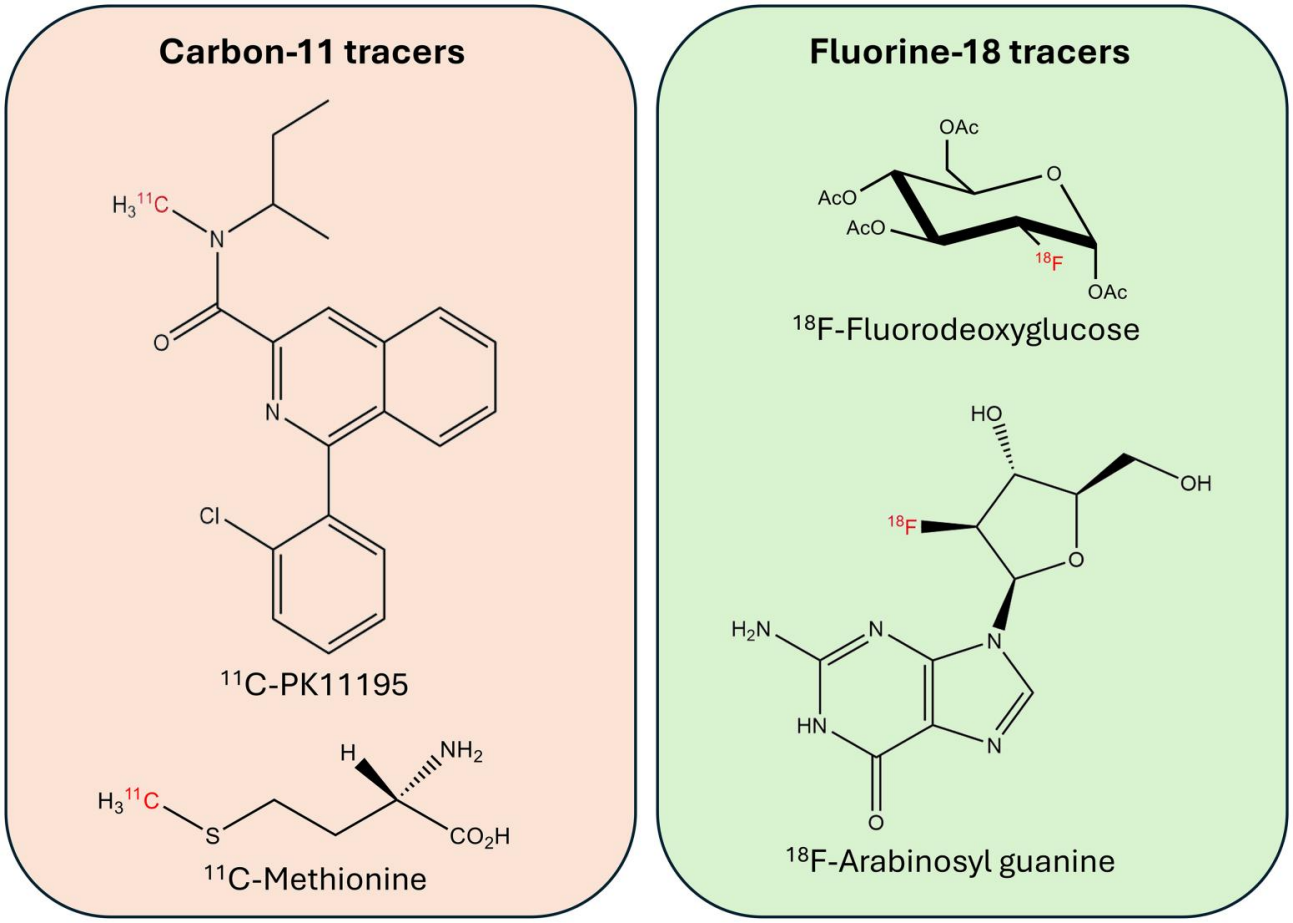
Examples of carbon-11 (^11^C) and fluorine-18 (^18^F) tracers frequently used in PET imaging. ^11^C-PK11195 targets the translocator protein (TSPO) and has been used to image activated microglia, which are involved in neuroinflammatory processes. It has applications in studying neuroinflammation in diseases like multiple sclerosis and Alzheimer's disease. ^11^C-methionine primarily targets amino acid metabolism. It is used in imaging inflammatory cells that are actively synthesizing proteins. It is useful in studying immune cell activity in tumours and inflammatory lesions. ^18^F-fluorodeoxyglucose (^18^F-FDG) is the most widely used PET tracer in immunology and oncology. It highlights areas of high glucose uptake, such as inflammatory sites where immune cells (like macrophages, neutrophils and lymphocytes) are active. ^18^F-arabinosyl guanine (^18^F-AraG) targets deoxycytidine kinase (dCK). This tracer is used to image T-cell activation. It has been used in research to monitor the immune response, particularly in the context of cancer immunotherapy.
